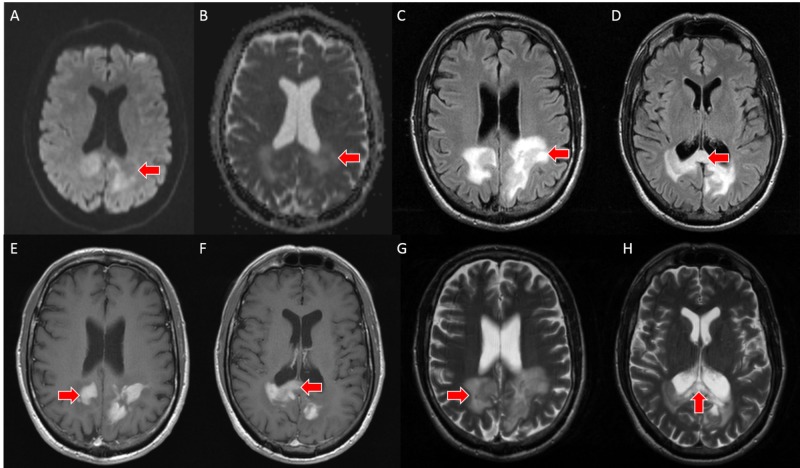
A - brain MRI with DWI sequence demonstrates mild restricted water diffusion associated with a mass in the bilateral parietal lobes and splenium (red arrow). B - brain MRI with ADC mapping demonstrates mild restricted water diffusion associated with a mass in the bilateral parietal lobes and splenium (red arrow). C, D - axial slices from brain MRI with FLAIR sequence demonstrates perilesional vasogenic edema within the splenium of the corpus callosum and in the adjacent parietal lobes (red arrows). E, F - axial slices from brain MRI with T1-weighted post-contrast sequence demonstrate discontinuous, homogeneous enhancement (red arrows). G, H - axial slices from brain MRI with T2 sequence demonstrate an isointense mass in the splenium of the corpus callosum and in the adjacent parietal lobes with surrounding vasogenic edema (red arrows) MRI: magnetic resonance imaging, DWI: diffusion-weighted imaging, ADC: apparent diffusion coefficient, FLAIR: fluid-attenuated inversion recovery.
